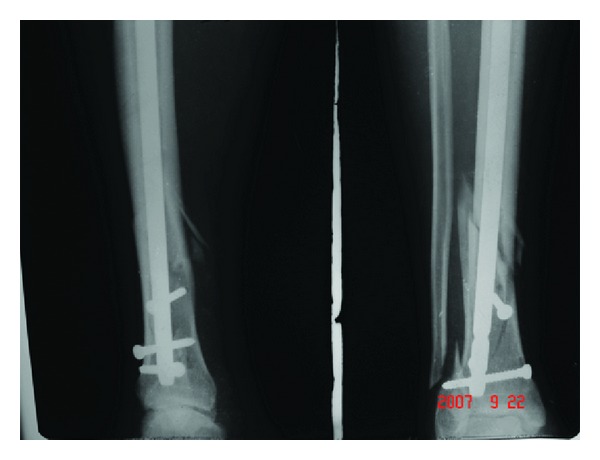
Immediate postoperative X-ray showing good alignment in spite of additional fracture line due to poller screw.
